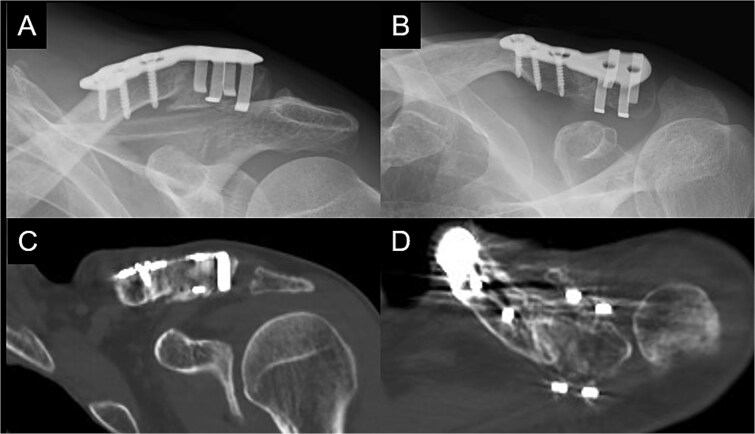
Radiographs (A and B) and CT scans (C and D) captured at 1 year postoperatively show union of the bone.
